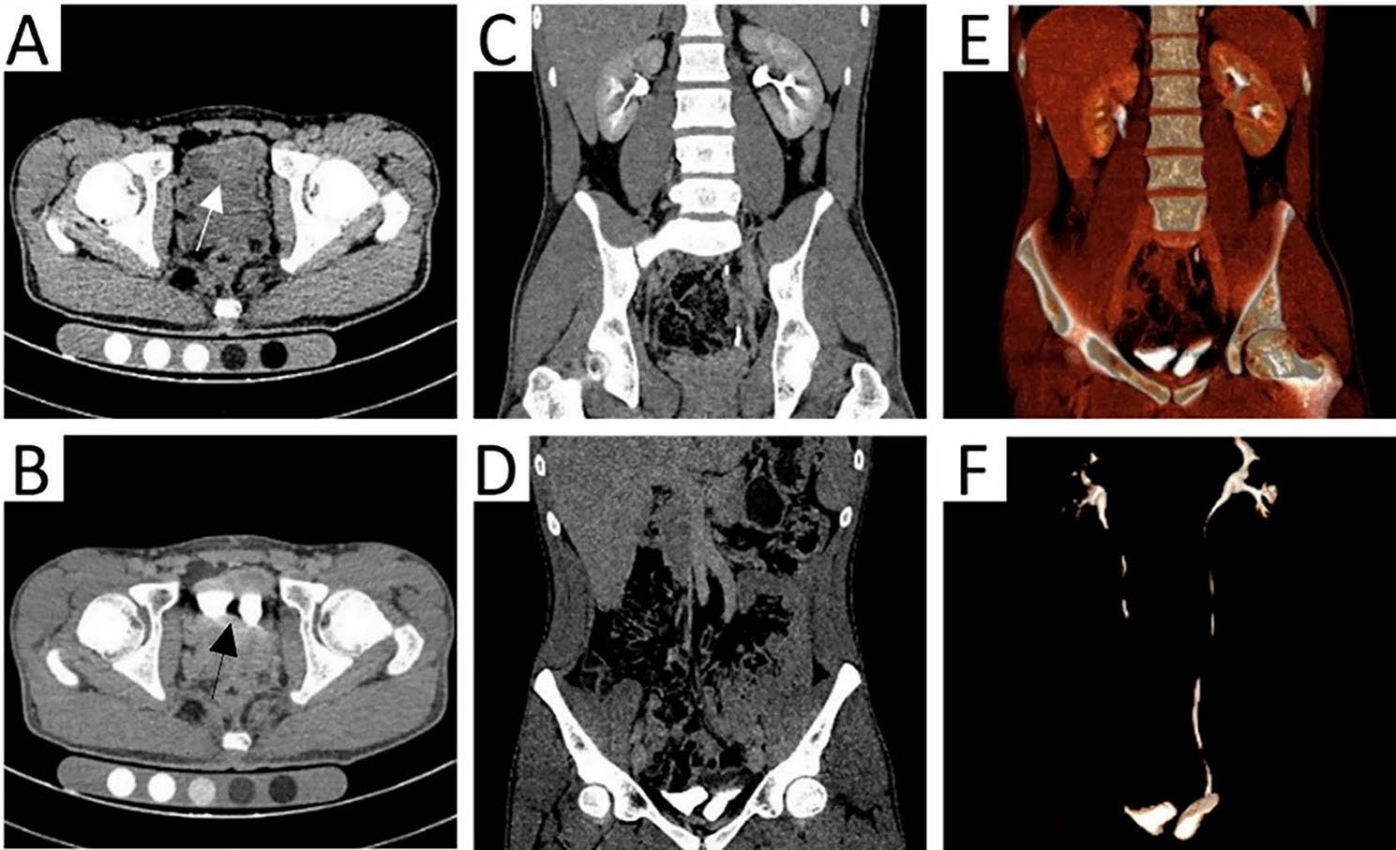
Urinary CT: cross-sectional images: a separation of seven millimeters (white and black arrow) in the bladder (A-B), there are two contrasts filled chambers in the pelvic cavity. MIP coronal view: the left and right kidneys were connected to each side of an independent ureter, and the two bladder cavities were side by side (C-E). VR: the entire urinary system can be seen. The left and right kidneys and a single ureter connect the left and right bladders (F)
